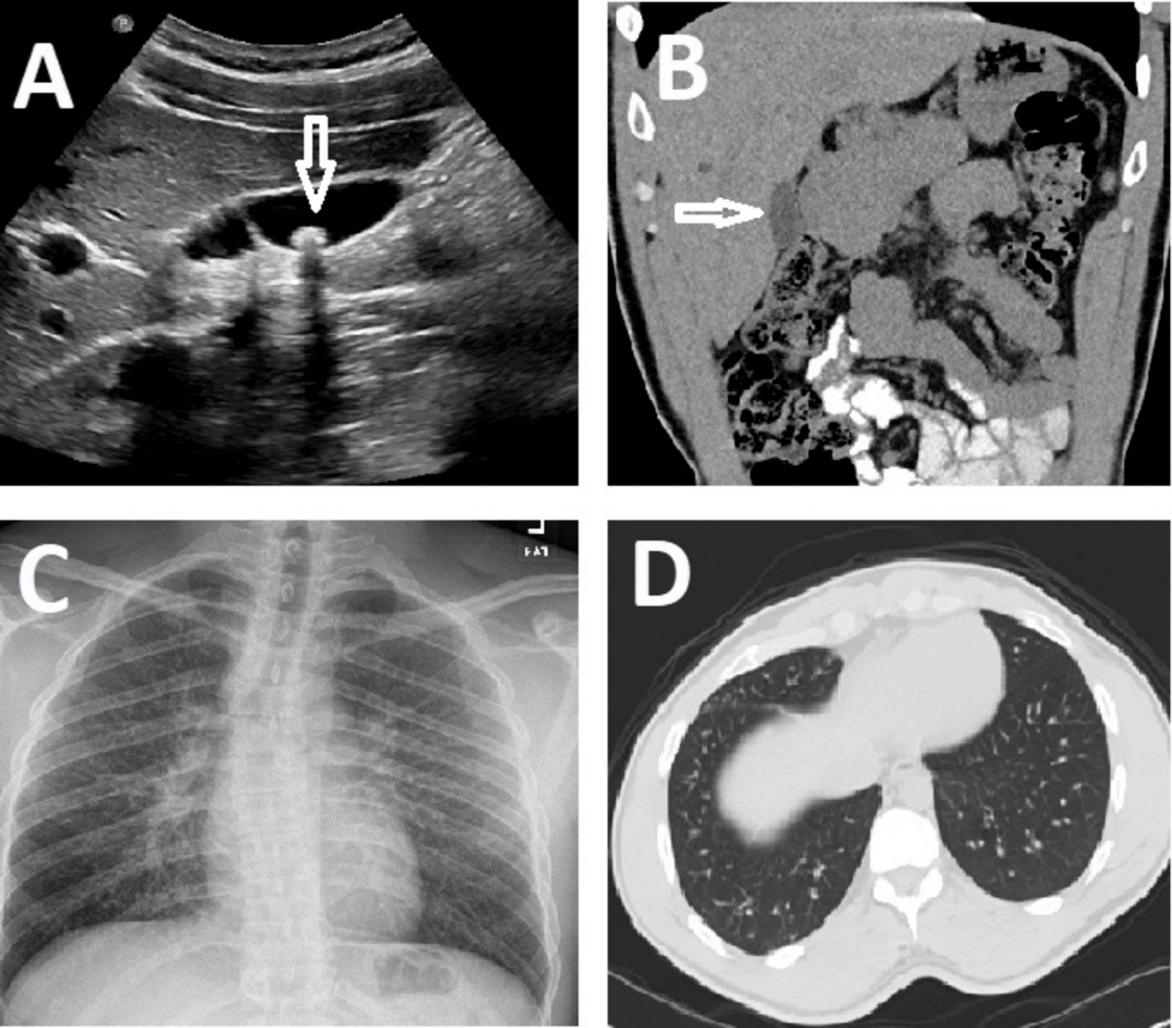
Multimodal Imaging Findings (A) Sagittal ultrasound image of the gallbladder representative of a hyperechoic gallstone with posterior acoustic shadowing (arrow). (B) Representative coronal CT image showing no evidence of acute cholecystitis or pericholecystic fluid (arrow). (C) CXR image with no overt pathologies. (D) Representative axial CT image showing mild airway thickening in the lung bases. CT: computed tomography, CXR: chest X-ray
